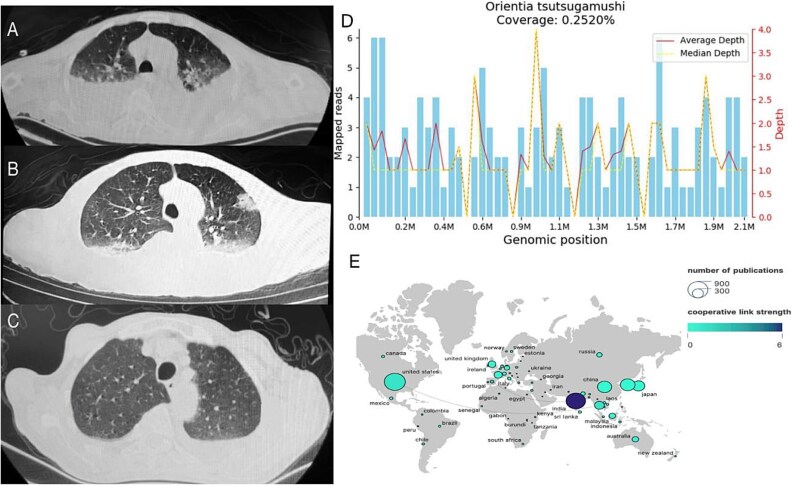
Chest CT examination, mNGS sequencing results and visual map of studies on tsutsugamushi in different countries.(A) on November 7, a high resolution chest CT showed double lung pneumonia, moderate pleural effusion and partial atelectasis. (B) on November 11, chest CT examination revealed double lung pneumonia and decreased pleural effusion. (C) on November 22, chest CT examination again showed that the pneumonia infiltration was significantly absorbed and the pleural effusion was significantly less than before. (D)analysis results of mNGS sequencing. The coverage of referenced *Orientia tsutsugamushi* genome was 0.25%. (E) visual maps of international cooperation between countries studying *O. tsutsugamushi*. 8442 items were found in the web of science using keywords like "scrub typhus", "tsutsugamushi fever", "*O. tsutsugamushi*", "tsutsugamushi disease", "tropical typhus", "epidemic typhus", and "tsutsugamushi pneumonia".
